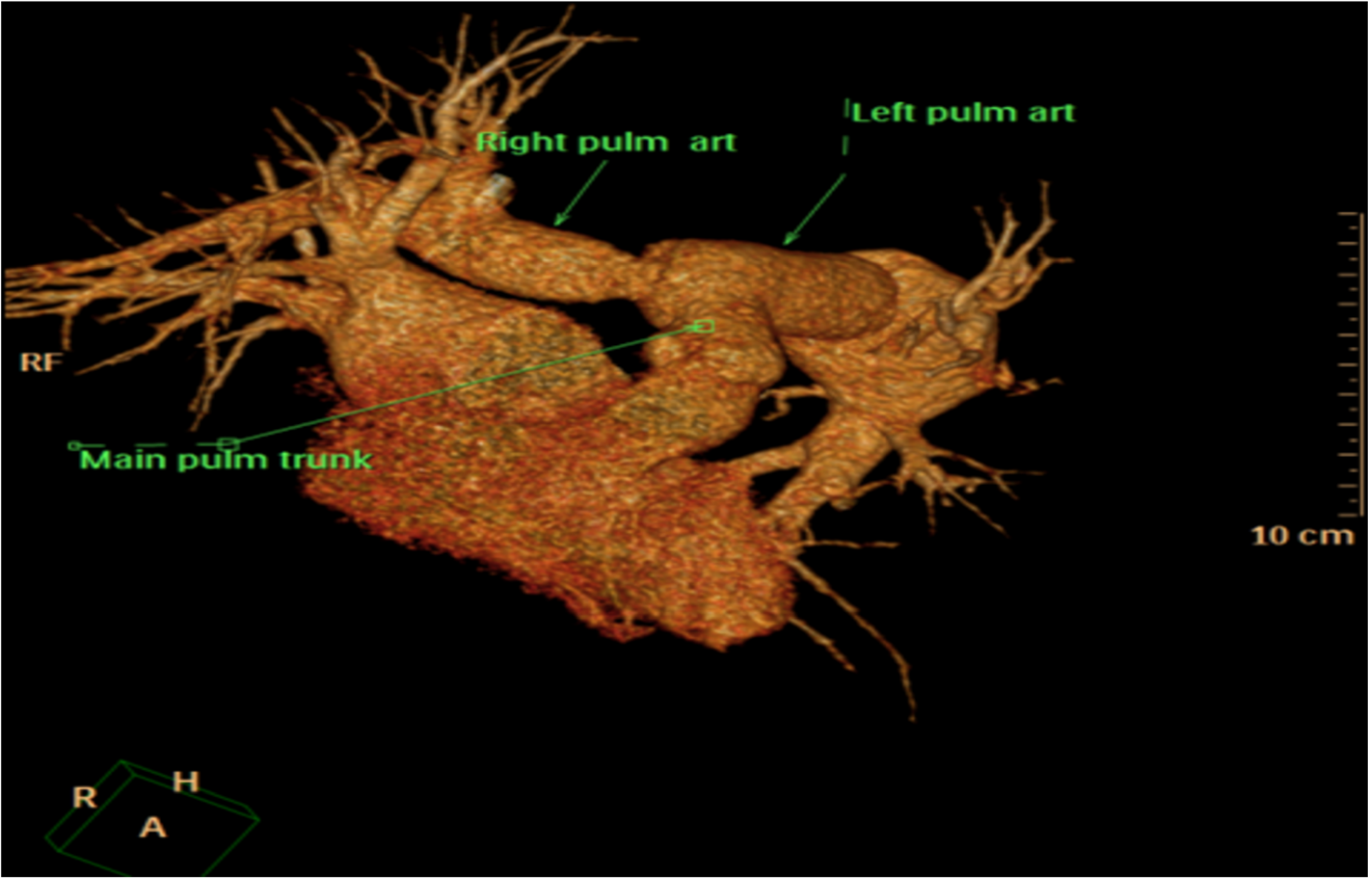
3D - Chest computed tomography angiogram scan (CT) showing: Pulmonary artery trunk, left pulmonary artery and right pulmonary artery replaced by Wovex tube graft without dilatation in the great vessels or stenosis
